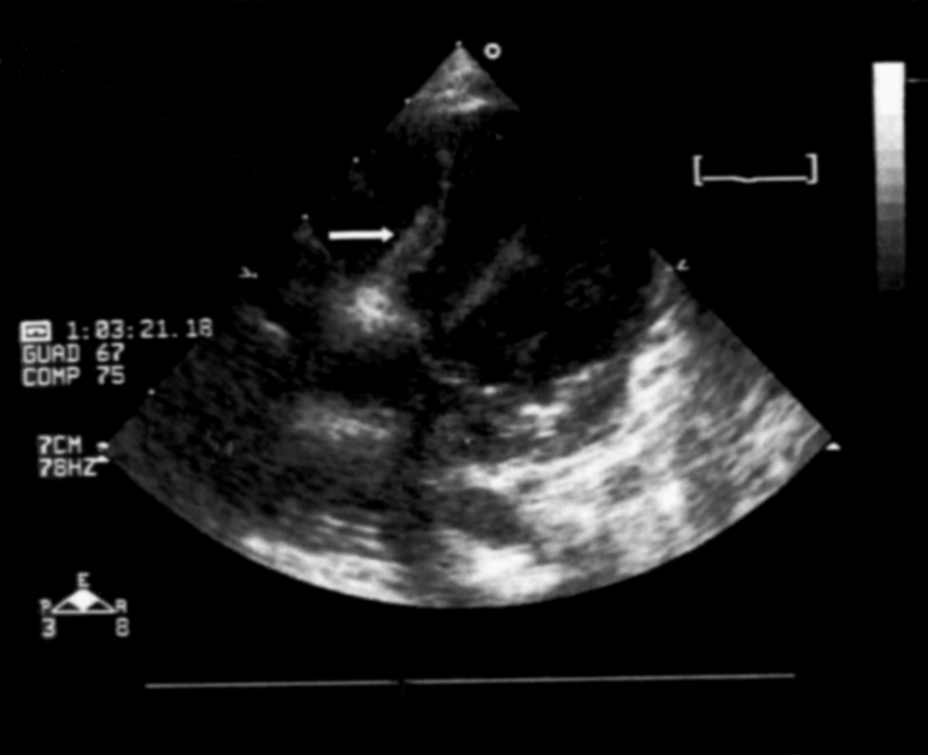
Echocardiographic apical four-chamber view. Bowel loops severely compressing the right side of the heart (white arrow).
